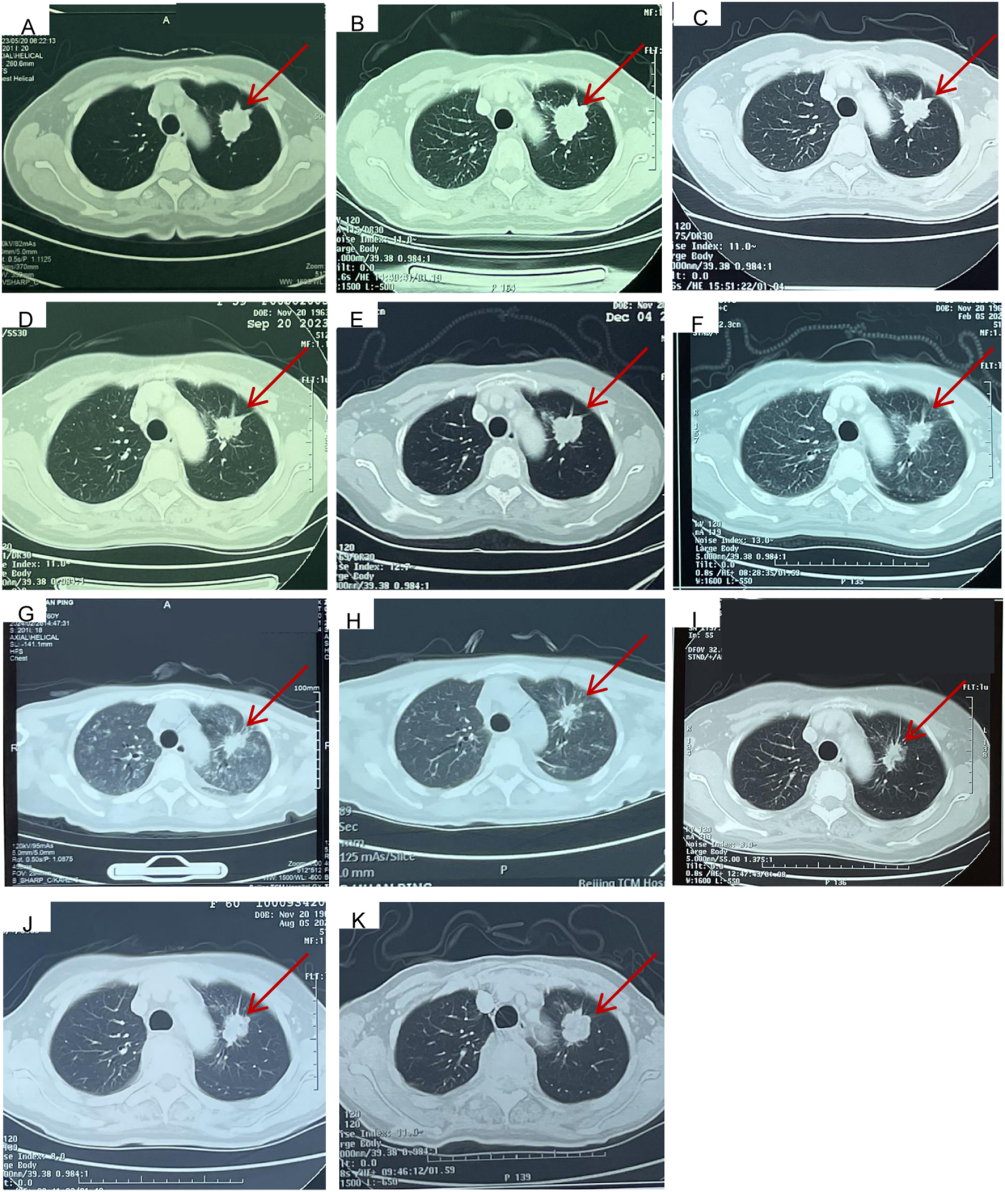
Computed tomography findings. (A) Primary lesion (26*37mm) in upper left lung at the time of first visit to Cancer Hospital, Chinese Academy of Medical Sciences on May, 20, 2023. (B) Baseline image (Primary lesion 29*36mm) before taken front-line pemetrexed and carboplatin chemotherapy along with bevacizumab targeted therapy at Jun, 15, 2023. (C) Response evaluation (Primary lesion 29*22mm, SD) after taken 2 cycles of pemetrexed and carboplatin chemotherapy along with bevacizumab targeted therapy at Jul, 24, 2023. (D) Response evaluation (Primary lesion 29*22mm, SD) after taken 4 cycles of pemetrexed and carboplatin chemotherapy along with bevacizumab targeted therapy at Sept, 20, 2023. (E) Twenty-one days after taken almonertinib combined with glumetinib targeted therapy (Primary lesion 30*28mm, SD) at Dec, 5, 2023. (F) eighty-four days after taken almonertinib combined with glumetinib targeted therapy (Primary lesion 26*18mm, PR) at Feb, 5, 2024. (G) The emergency chest CT indicated diffuse interstitial lung disease (ILD) at Feb, 26, 2024, (Primary lesion 28*20mm, SD). (H) The ILD has been well controlled at Mar, 4, 2024, (Primary lesion 28*20mm, SD). (I) twenty-two days after the rechallenge of the dual-targeted therapy at May, 7, 2024, (Primary lesion 28*19mm, SD). (J) Response evaluation (Primary lesion 28*19mm, SD) at Aug, 5, 2024. (K) Response evaluation (Primary lesion 28*25mm, SD, but showing a slow progress trend) at Nov, 11, 2024.
